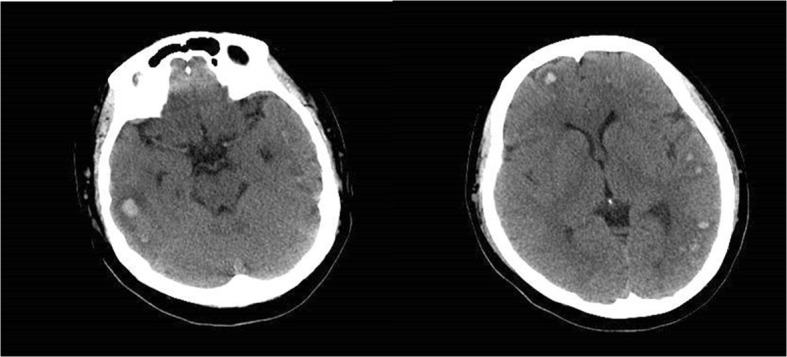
Plain head computed tomography conducted on day 7 of the patient’s hospital stay showing multiple intracranial hemorrhages
